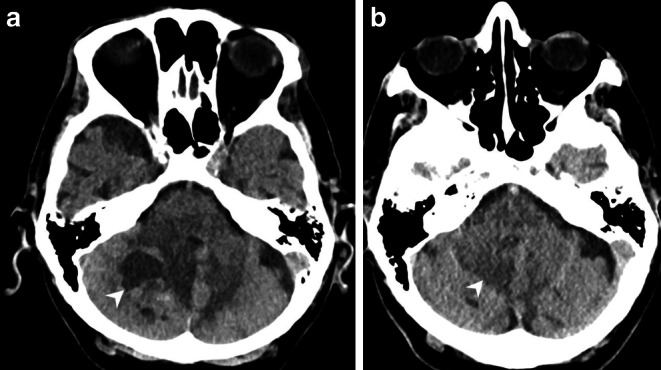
Axial cranial CT images at admission (a) in soft tissue window showed a well-circumscribed mass lesion located in the right cerebellar hemisphere (*arrowhead*). The patient had another cranial CT 3.5 years prior to admission (b). In retrospect, the lesion was already detectable (*arrowhead*) but appeared much smaller
